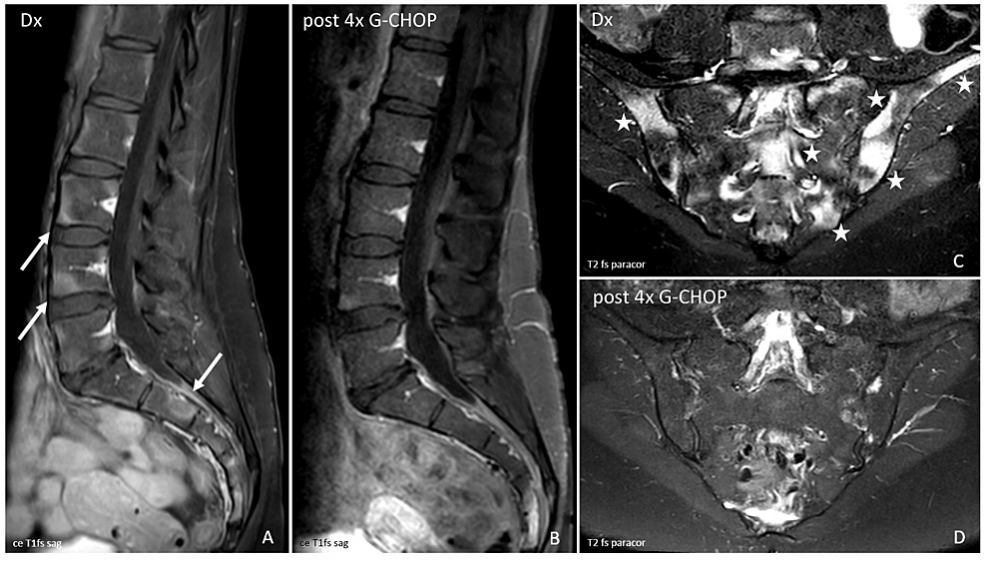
Magnetic resonance imaging Contrast-enhanced T1 fat-saturated (fs)-sequence with sagittal slides (ce T1 fs sag) of the lower thoracic spine, lumbar spine, and sacral bone (A, B) and T2 fs-sequence with paracoronal slides (T2 fs paracor) of the sacroiliac joints (SIJ) (C, D). Intense contrast uptake of lesions in the area of the entheses of the vertebral body endplates and the sacral bone (arrows in A). Bone marrow edema is represented by T2-hyperintense lesions in the medullary cavity of the pelvis (massae laterales) in contact with the articular surfaces of the SIJ and remote from the joint in the os ilium (stars in C). Partial remission of the lesions six months later after four cycles of G-CHOP (post 4x G-CHOP) (B, D). Dx = imaging at first diagnosis; G-CHOP = obinutuzumab plus cyclophosphamide, doxorubicin, vincristine, and prednisone.
